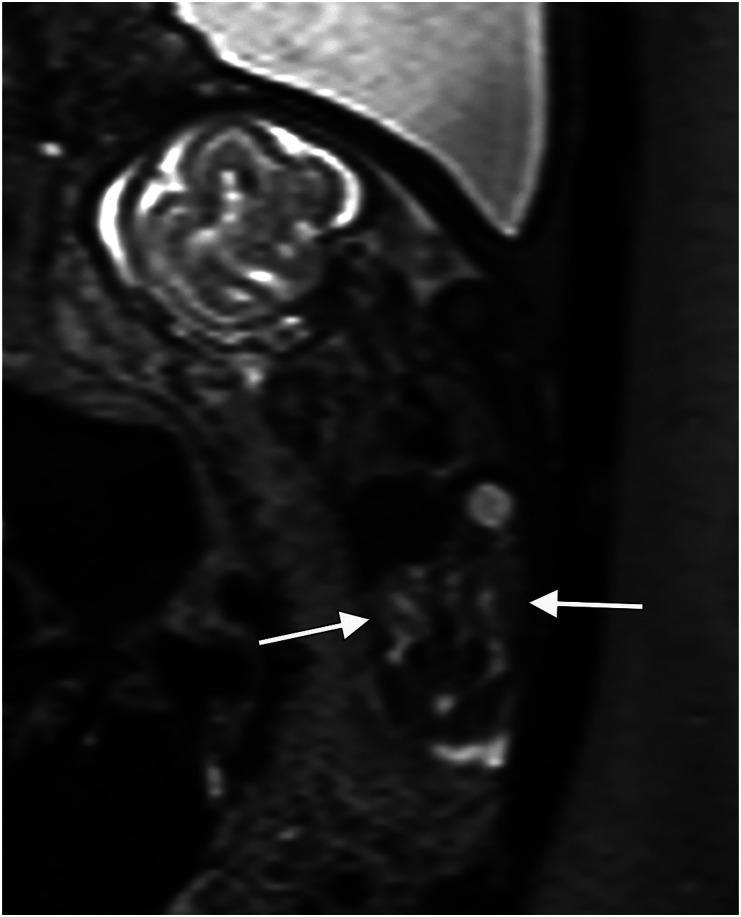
Case no 31. At ultrasound examination, there was a suspicion of polycystic kidney disease. Coronal MR image shows kidneys of normal size and signal intensity (arrows).
